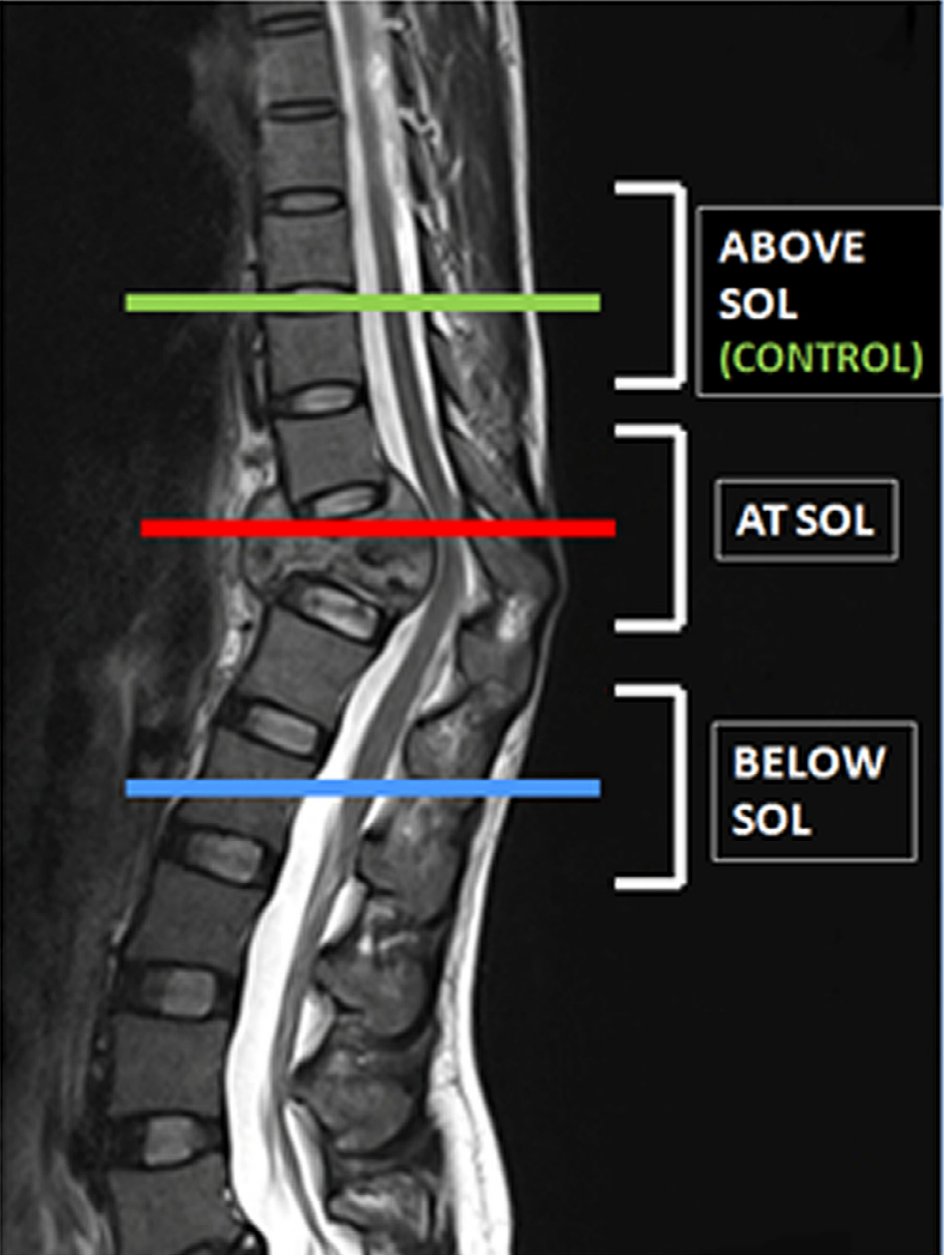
Levels of site of lesions (SOL) as marked on a T2 sagittal MRI image.
